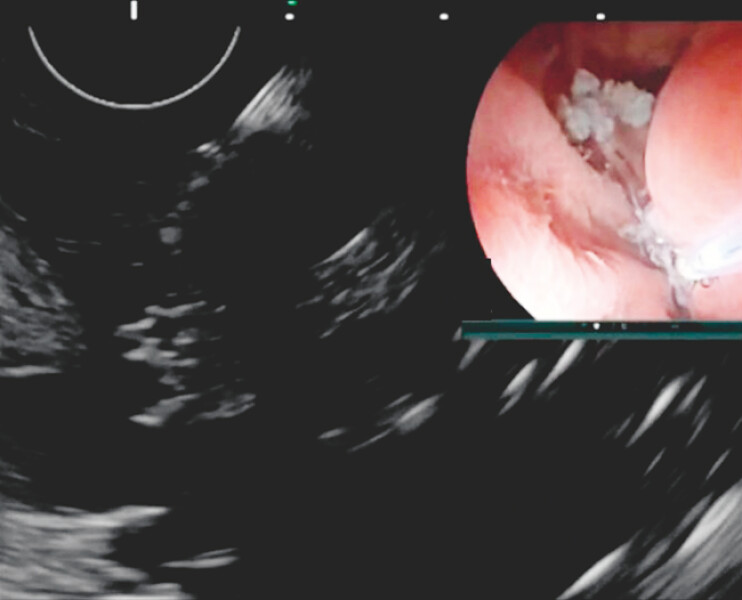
EUS was used to locate the pancreatic duct. The cauterization marking was performed under EUS guidance. Abbreviation: EUS, endoscopic ultrasound.
